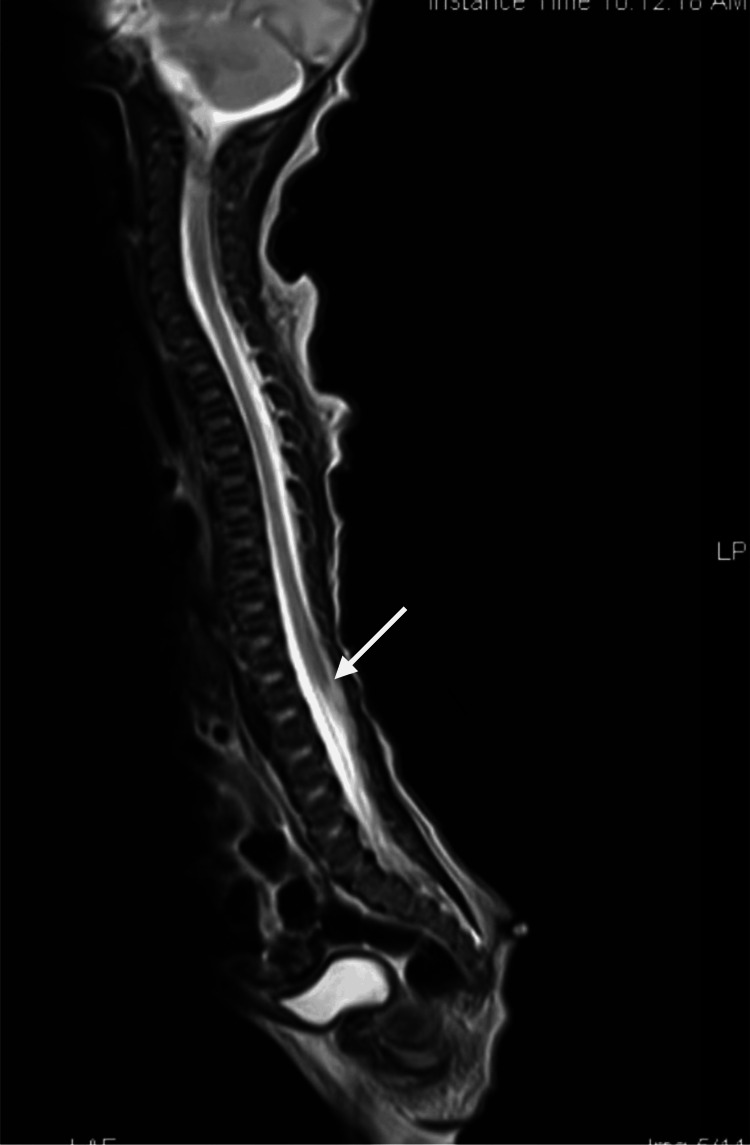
MRI of the spine shows the normal end level of the conus medullaris (arrow)
